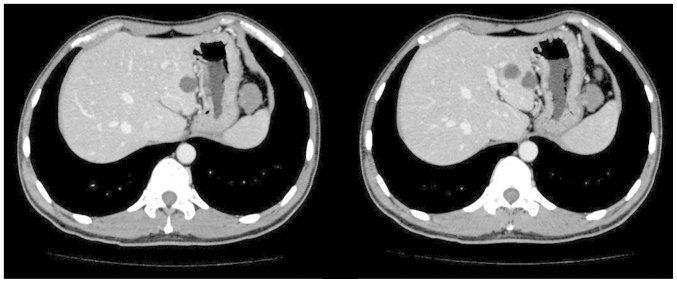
A mass with a maximum diameter of ~2.8 cm and a CT value of ~36 Hounsfield units was identified in the left upper quadrant (left). It appeared that the mass was originating from the body of the stomach (right), and a gastric stromal tumor was suspected due to the CT scans.
